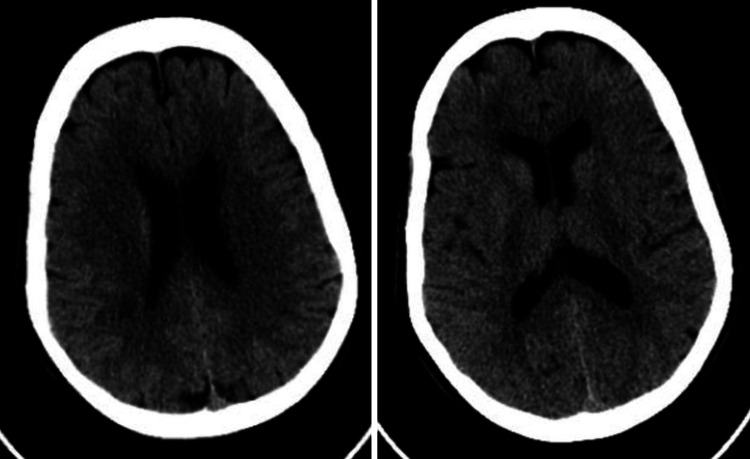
Unenhanced CT brain showing few focal hypodensities involving the right frontal and right parietal lobes
